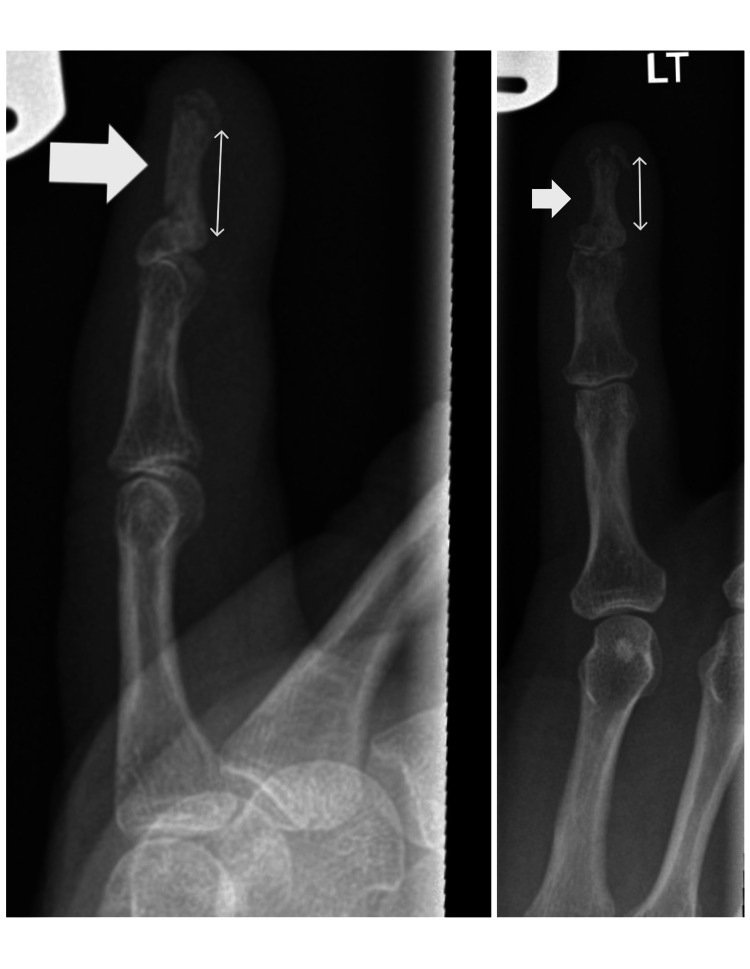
Follow-up antero-posterior and lateral radiographs at six weeks demonstrating bony union
